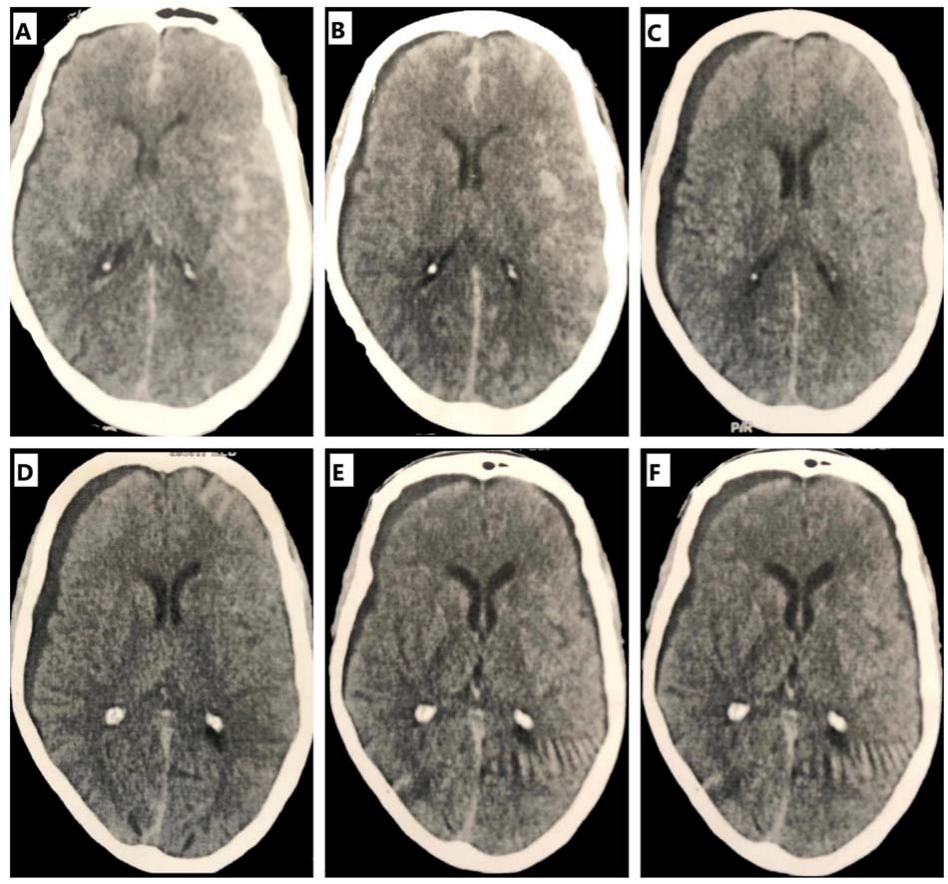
Serial unenhanced axial CT scans of the brain. (A) First CT after coiling at day 2. (B) At day 3, showing right subdural hygroma on the opposite side of leaking aneurysm. (C) At day 5, showing increased hygroma and no signs of ventriculomegaly. (D) At day 10, showing more increased hygroma and mild mass effect. (E) At day 14, showing newly developed left small hygroma. (F) At day 30, showing the regressing right hygroma.
